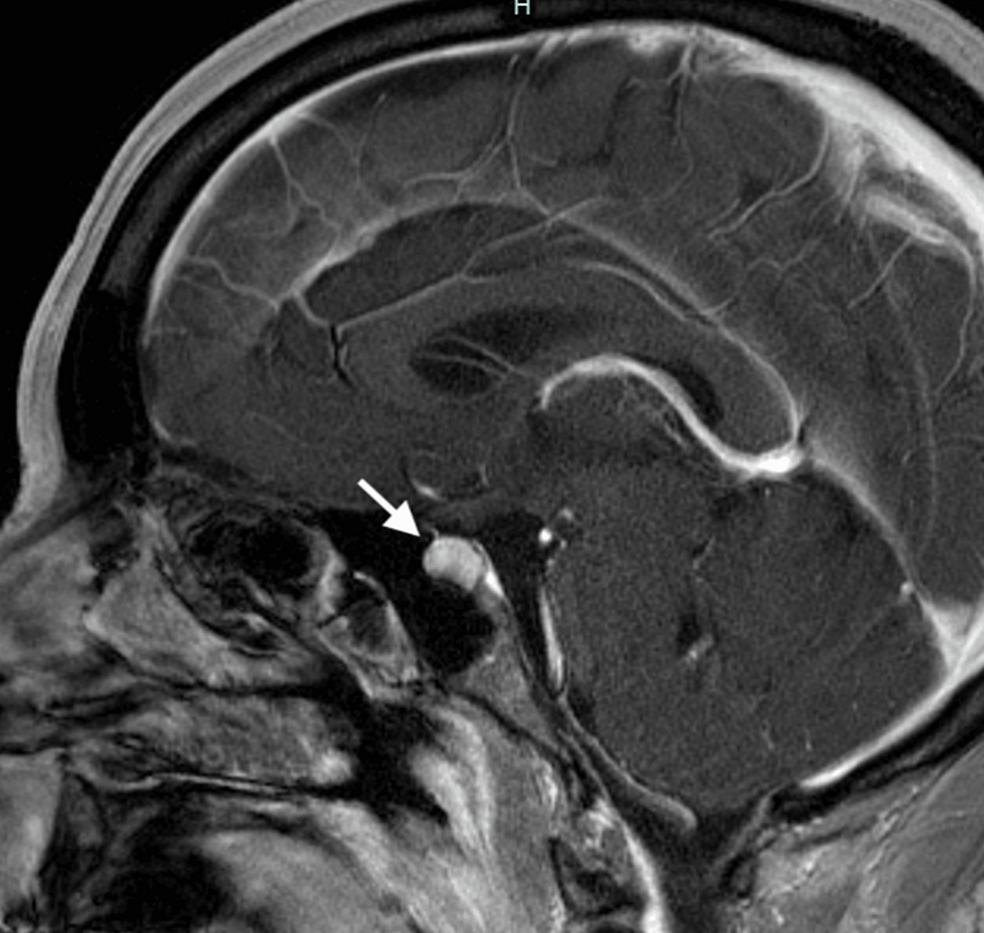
MRI of the brain and pituitary showing prominence of the pituitary gland with heterogenous enhancement.
